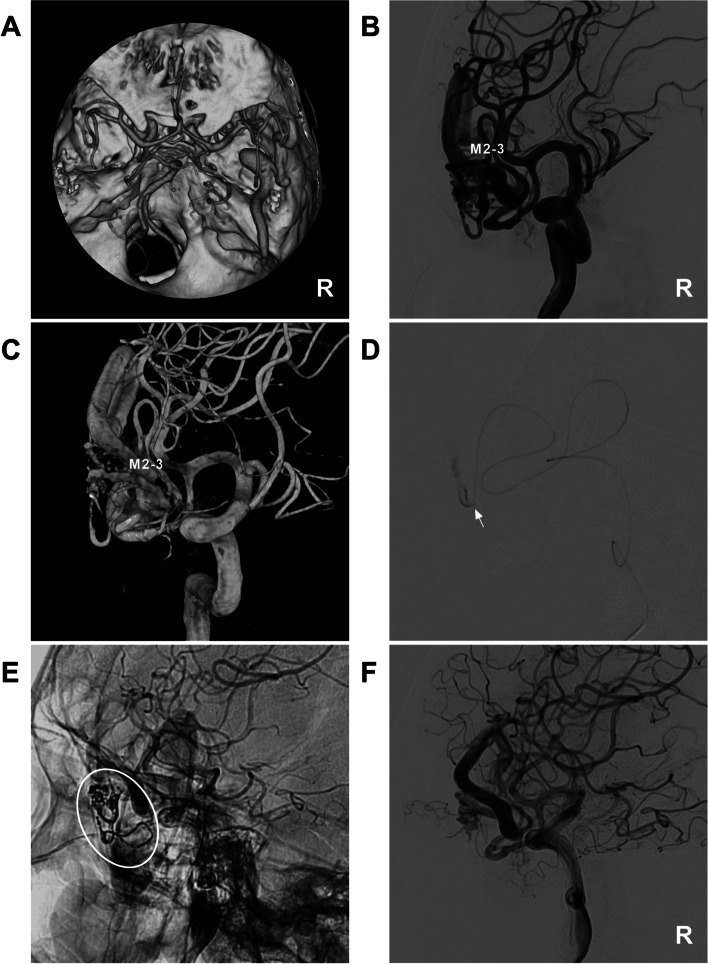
EVT of a type II BAVM. A CTA shows a BAVM located in the right Sylvian fissure, and the draining vein is varicose. B-C Two-dimensional angiogram (B) and three-dimensional reconstruction angiogram of the right ICA (C) show that the BAVM is supplied by the branches of the M2-3 segments, and the draining vein is varicose. D Superselective angiogram shows that the wedged position has been achieved for the microcatheter (arrow). E Unsubtracted angiogram showing casting Onyx (circle). F: Right ICA angiogram shows that the volume of the BAVM nidus is reduced after EVT, and the draining vein is patent. Abbreviations: BAVM: brain arteriovenous malformation, CTA: computed tomography angiography, EVT: endovascular treatment, ICA: internal carotid artery, MCA: middle cerebral artery, M2-3: second and third segments of the MCA, R: right
